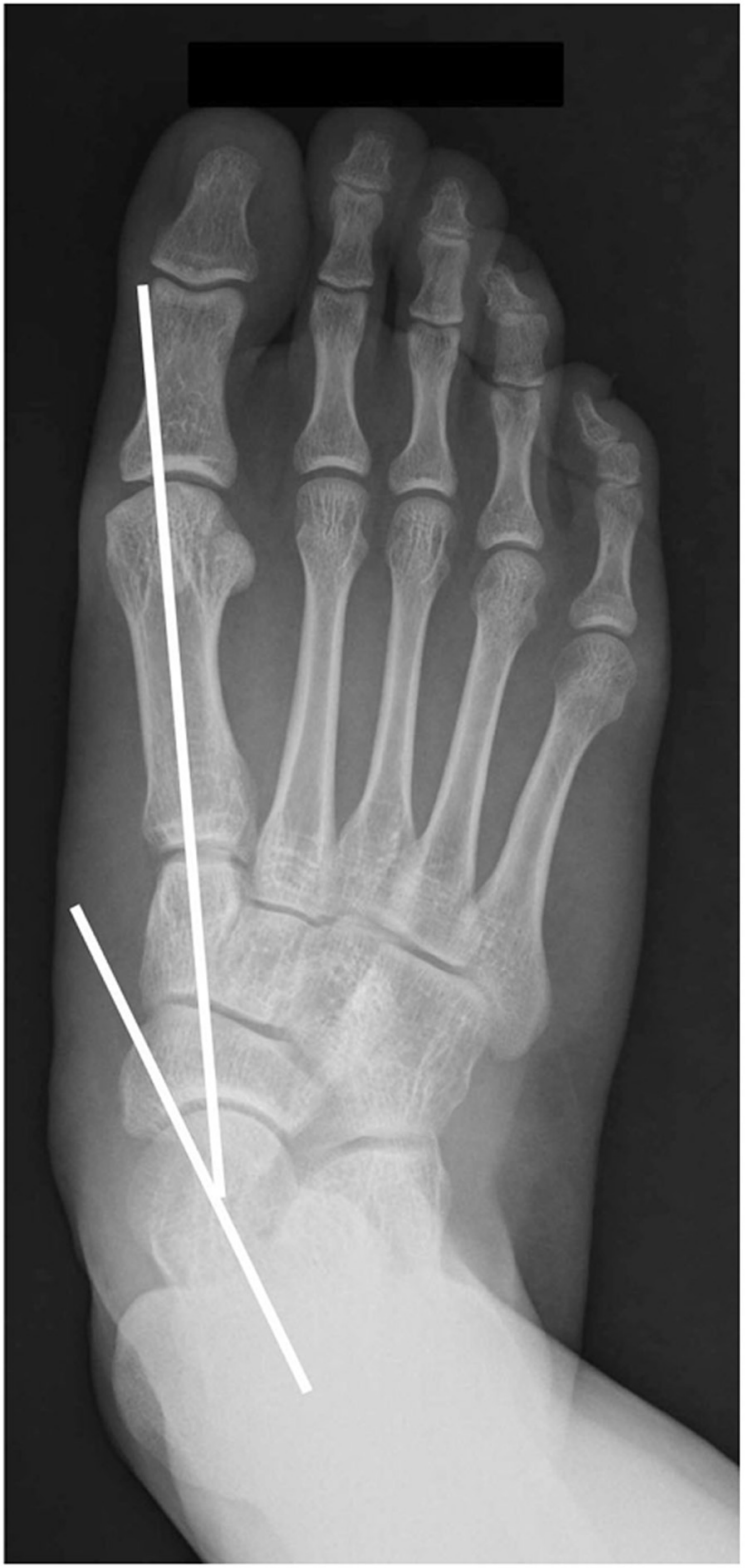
Talo-first metatarsal angle is the angle between the longitudinal axis of talus and that of first metatarsal bone on weight bearing anteroposterior foot radiograph.
